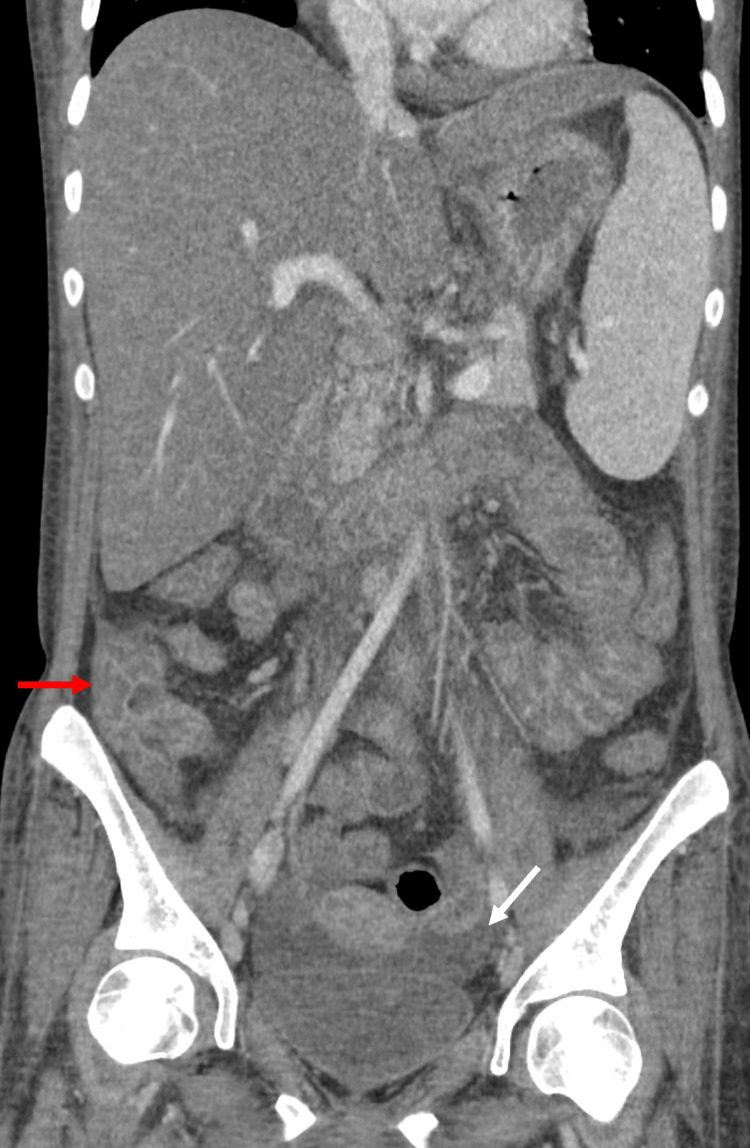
CT abdomen and pelvis coronal reformatted image demonstrating hepatosplenomegaly, diffuse colonic wall edema most pronounced in the cecum (red arrow), and pelvic free fluid (white arrow).
